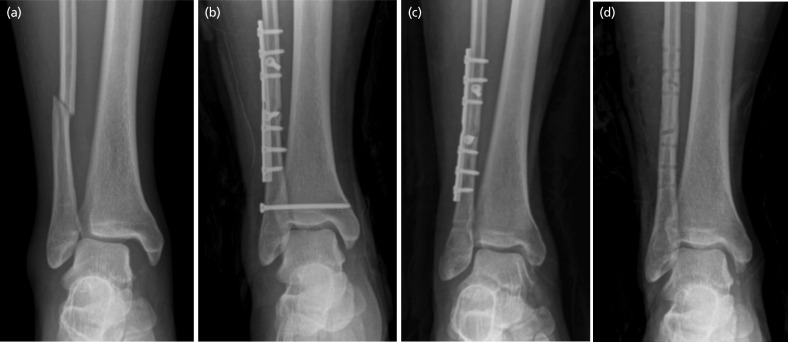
Static screw fixation of syndesmosis. (a) Pre-operative anteroposterior radiograph of right ankle showing supination external rotation-type ankle fracture. (b) The syndesmosis was fixed with a cortical screw. (c) At eight weeks post-operatively, the syndesmotic screw was removed. (d) At the one-year follow-up, all implants were removed; radiograph showing complete bone union and well-maintained ankle mortise.
